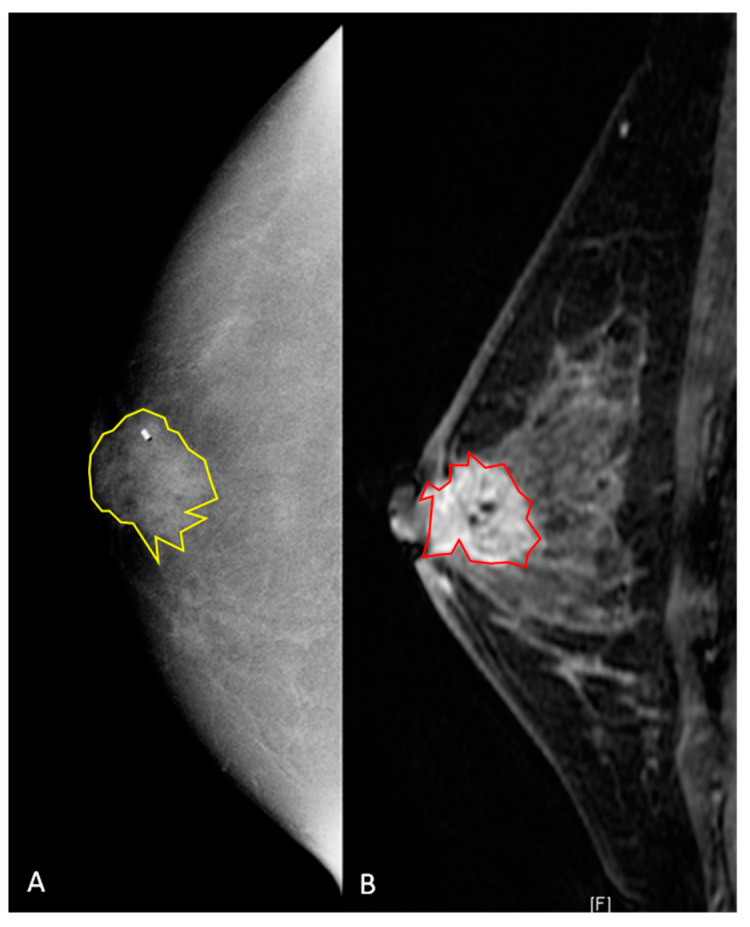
A 42-year old women with a biopsy-proven invasive ductal carcinoma (G3, HR positive, HER2 negative) in the right retro-areolar space. (A) Contrast-enhanced mammography shows, on the right, a 22 mm rounded area of enhancement. The lesion was manually segmented (2D yellow region of interest), and the clip marker had to be included in the segmented area. (B) Sagittal fat-saturated post-contrast enhanced T1-weighted image. The breast is heterogeneously dense with moderate background enhancement. In the retroareolar right breast, there is a 22 mm spiculated mass containing a localizing clip from biopsy. The mass is inseparable from the nipple, which appears slightly retracted, and there is associated skin thickening. The lesion was manually segmented (2D red region of interest).
